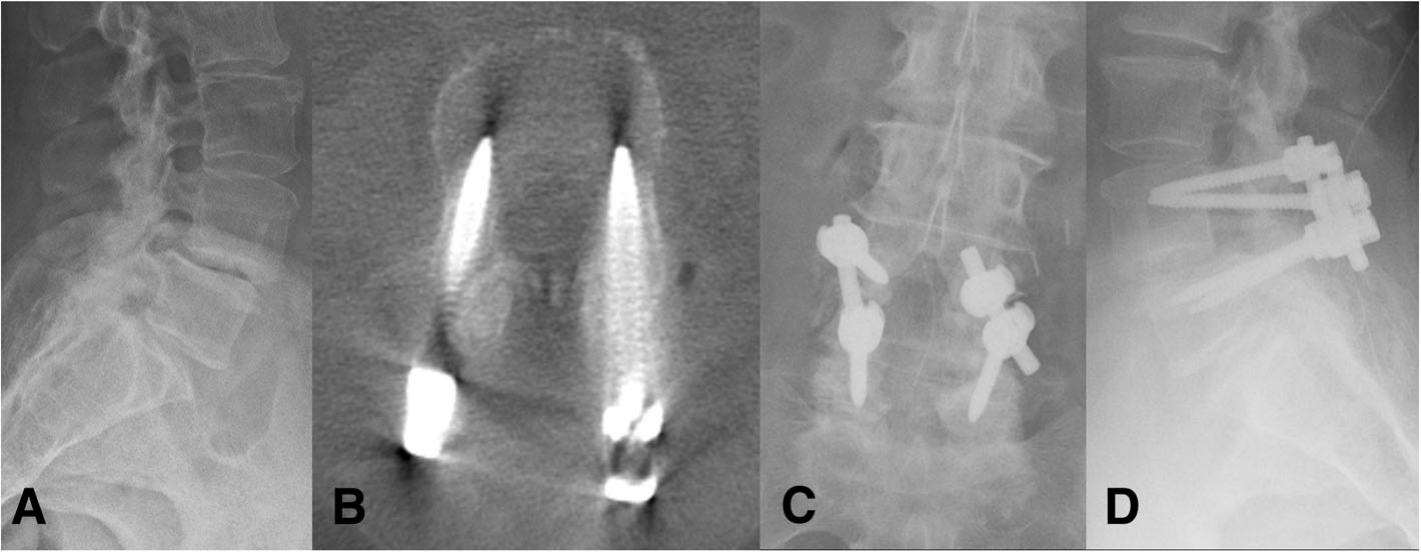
Representative case of a female who presented with radicular pain, weakness, and mechanical back pain due to a degenerative spondylolisthesis at L4/L5. a Lateral x-ray demonstrating degenerative spondylolisthesis at L4/L5. b Axial post-operative CT with ideal transpedicular screw placement. c Post-operative AP x-ray of L4/L5 instrumented arthrodesis. d Post-operative lateral x-ray
